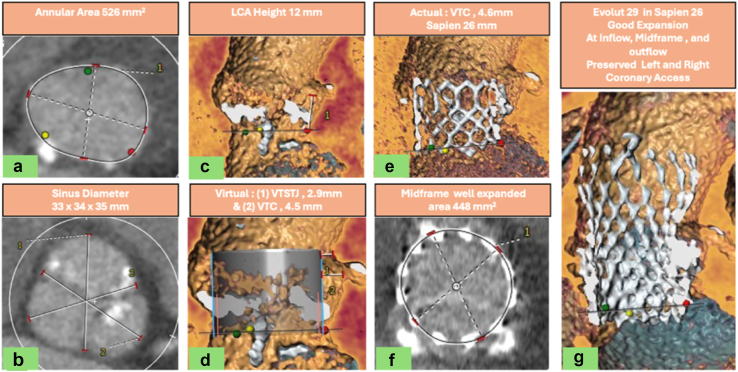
CT-basedassessment ofcoronary andsinotubularjunctionclearance forredo-TAVRfeasibility. Preprocedural CT demonstrated (a) an annular area of 526 mm^2^, (b) sinus of Valsalva dimensions of 33 × 34 × 35 mm, and (c) left coronary height of 12 mm. (d) Virtual modeling of a 26-mm balloon-expandable valve showed predicted preservation of the VTSTJ (2.9 mm) and VTC (4.5 mm). Postprocedural CT confirmed (e) an actual VTC of 4.6 mm after 26-mm Sapien implantation and (f) good post-TAVI midframe expansion area (448 mm^2^). (g) Redo-TAVR with a 29-mm self-expanding valve within the Sapien valve resulted in good expansion at the inflow, midframe, and outflow levels, with preserved bilateral coronary access and no coronary occlusion or sinus sequestration. Abbreviations: CT, computed tomography; LCA: left coronary height; TAVI, transcatheter aortic valve implantation; TAVR, transcatheter aortic valve replacement; VTC, valve-to-coronary distance; VTSTJ, valve-to-sinotubular junction distance.
